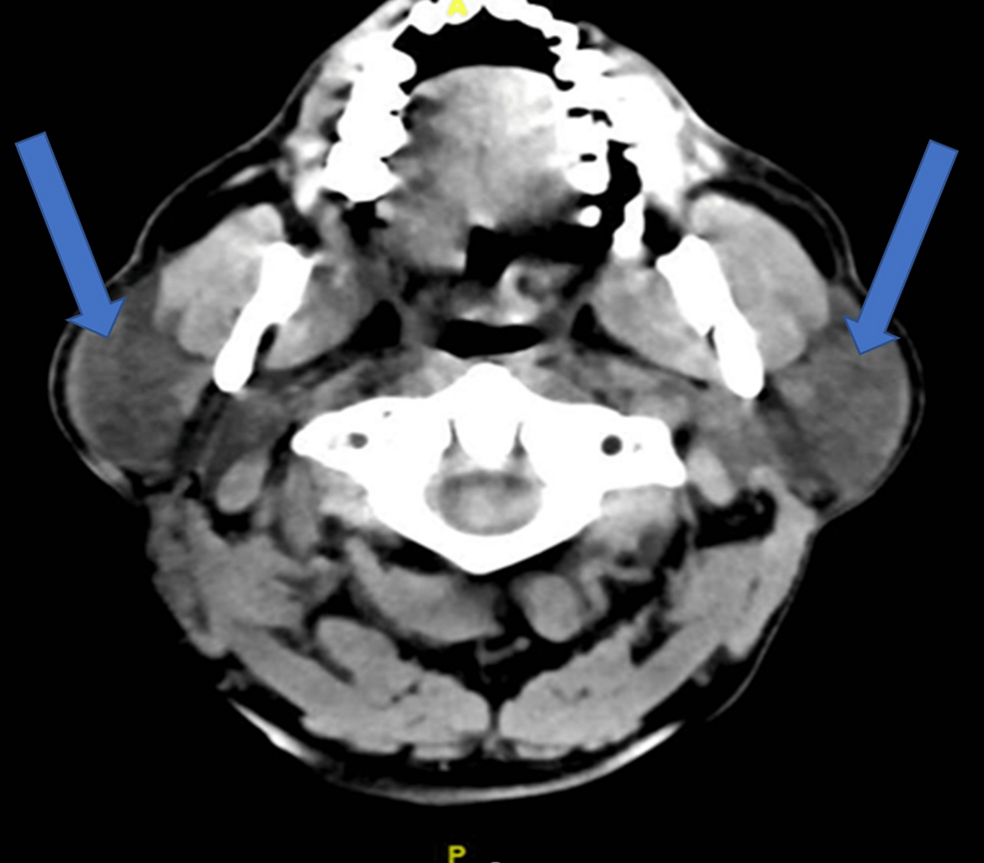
Brain CT showing bilateral parotid gland enlargement
